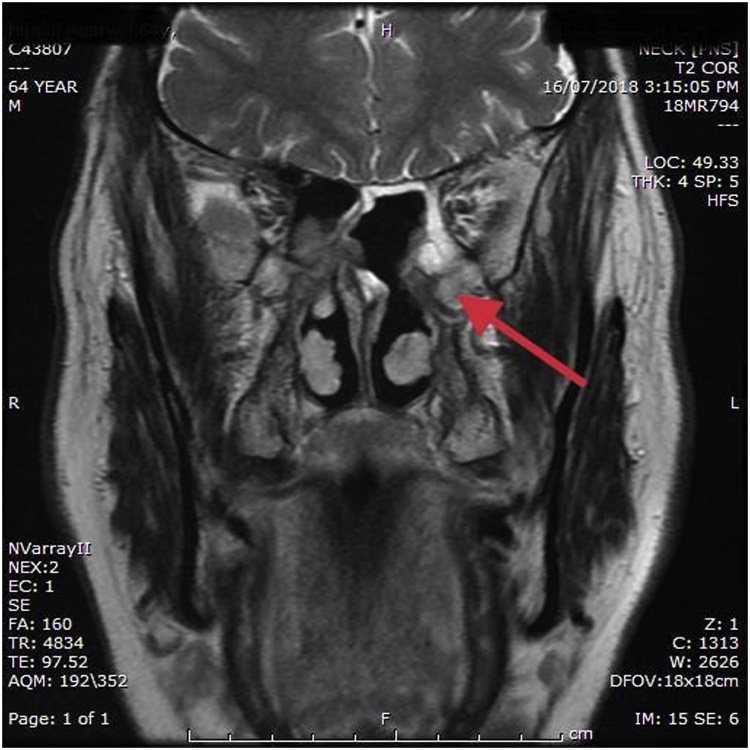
Post first surgery magnetic resonance imaging with contrast coronal section of paranasal sinus.
